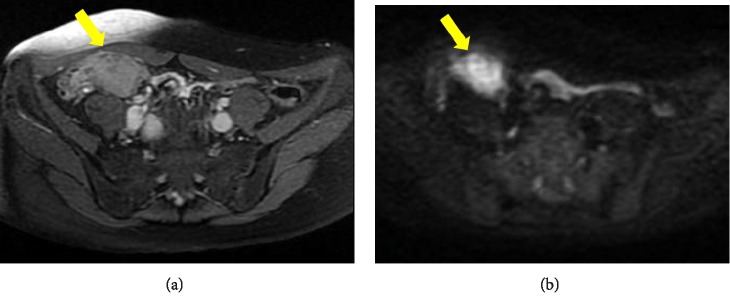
(a) MRI T2-weighted images showing mild high intensity lesions in the ileocecal region (arrow). (b) MRI diffusion-weighted images showing decreased diffusion in lesions (arrow).
